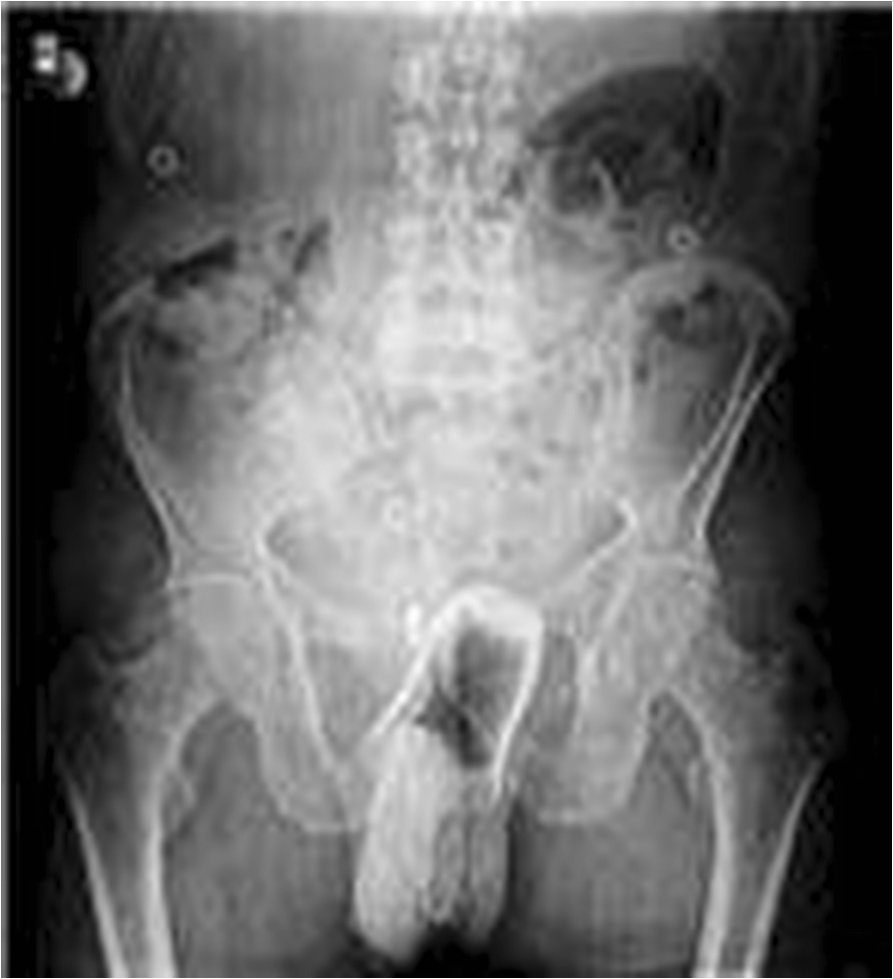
Rectal tea glass on abdominal plain film.
